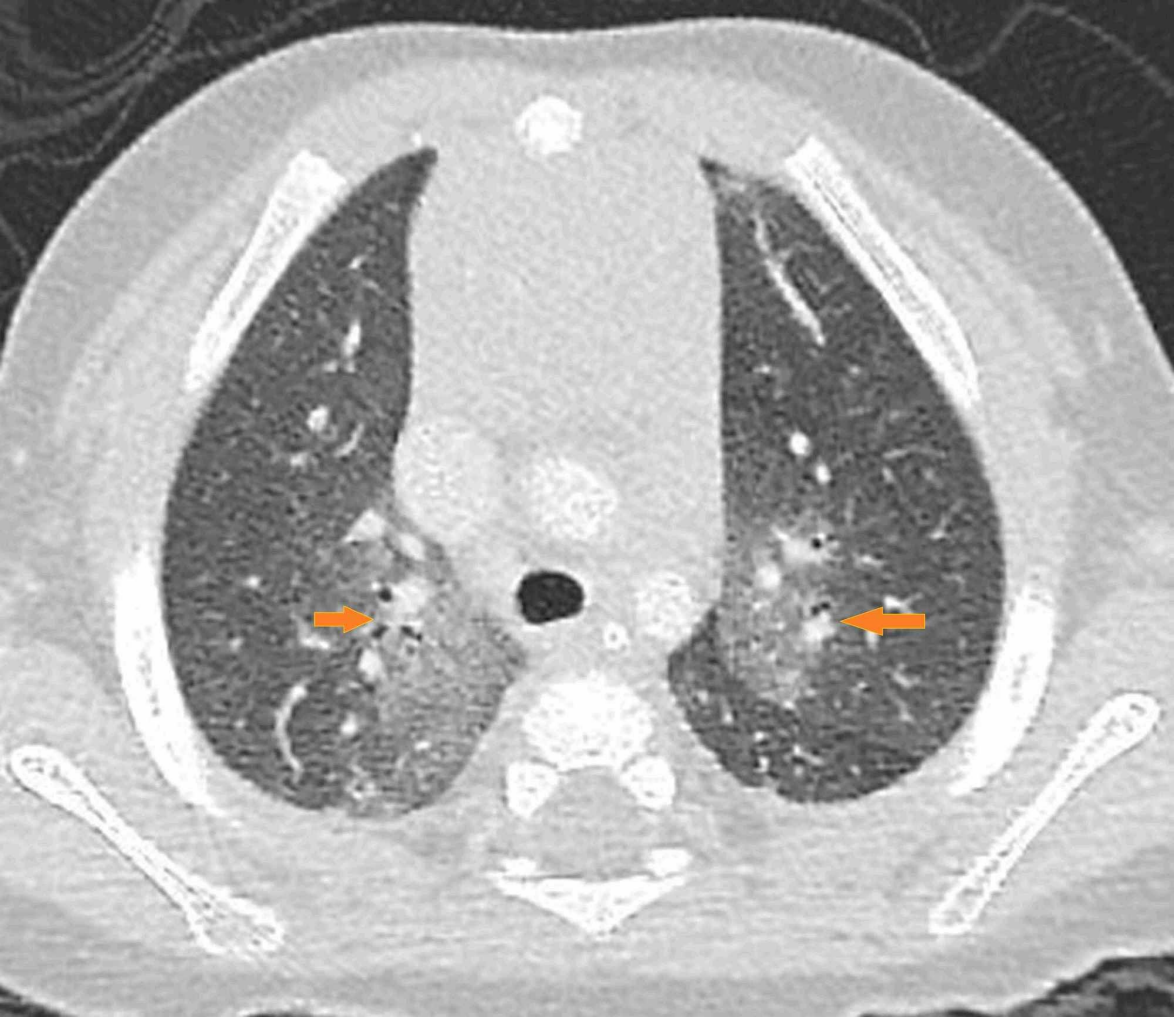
Axial HRCT section of the lung at the level of the upper lobe Arrows on both sides show the geographic ground-glass opacities. HRCT, high-resolution computed tomography
